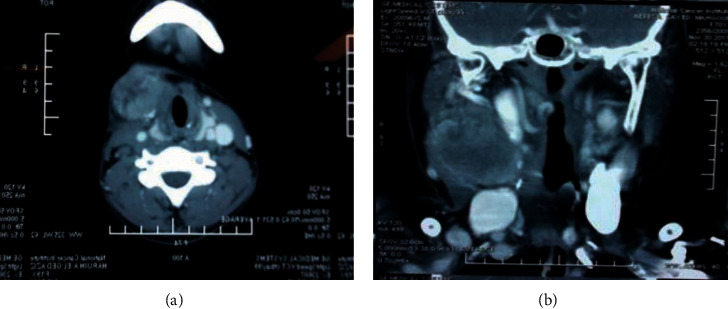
CT scan (axial and coronal views) for 2 patients showing right-sided neck mass diagnosed later as benign CBT.
